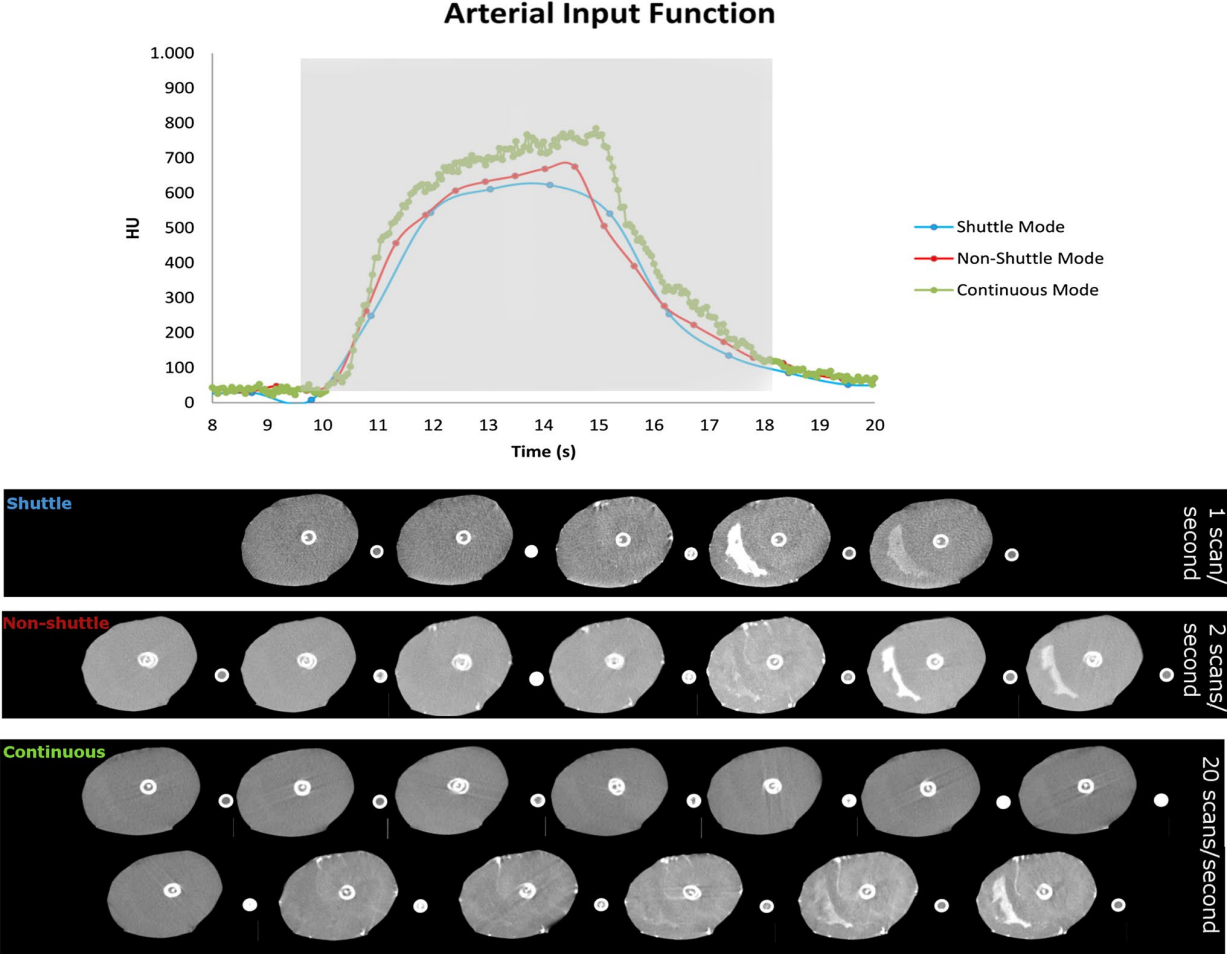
The arterial input functions (AIF) of shuttle (blue), non-shuttle (red) and continuous (green) perfusion mode with correlating CT images (Light grey square)
